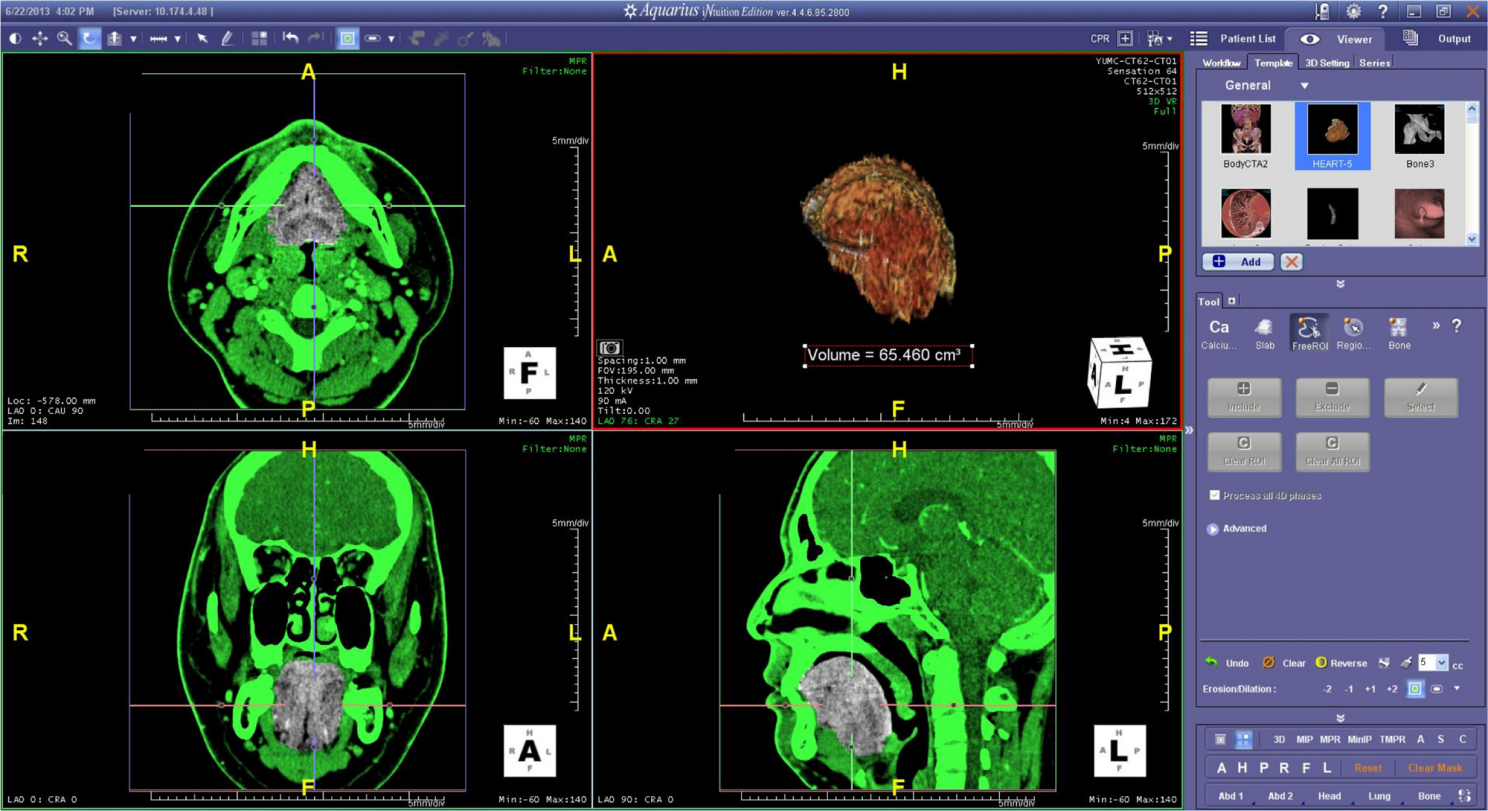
An example of 3D-reconstructed CT image of tongue and volume measurement by 3-D workstation software (Aquarius iNtuition).
